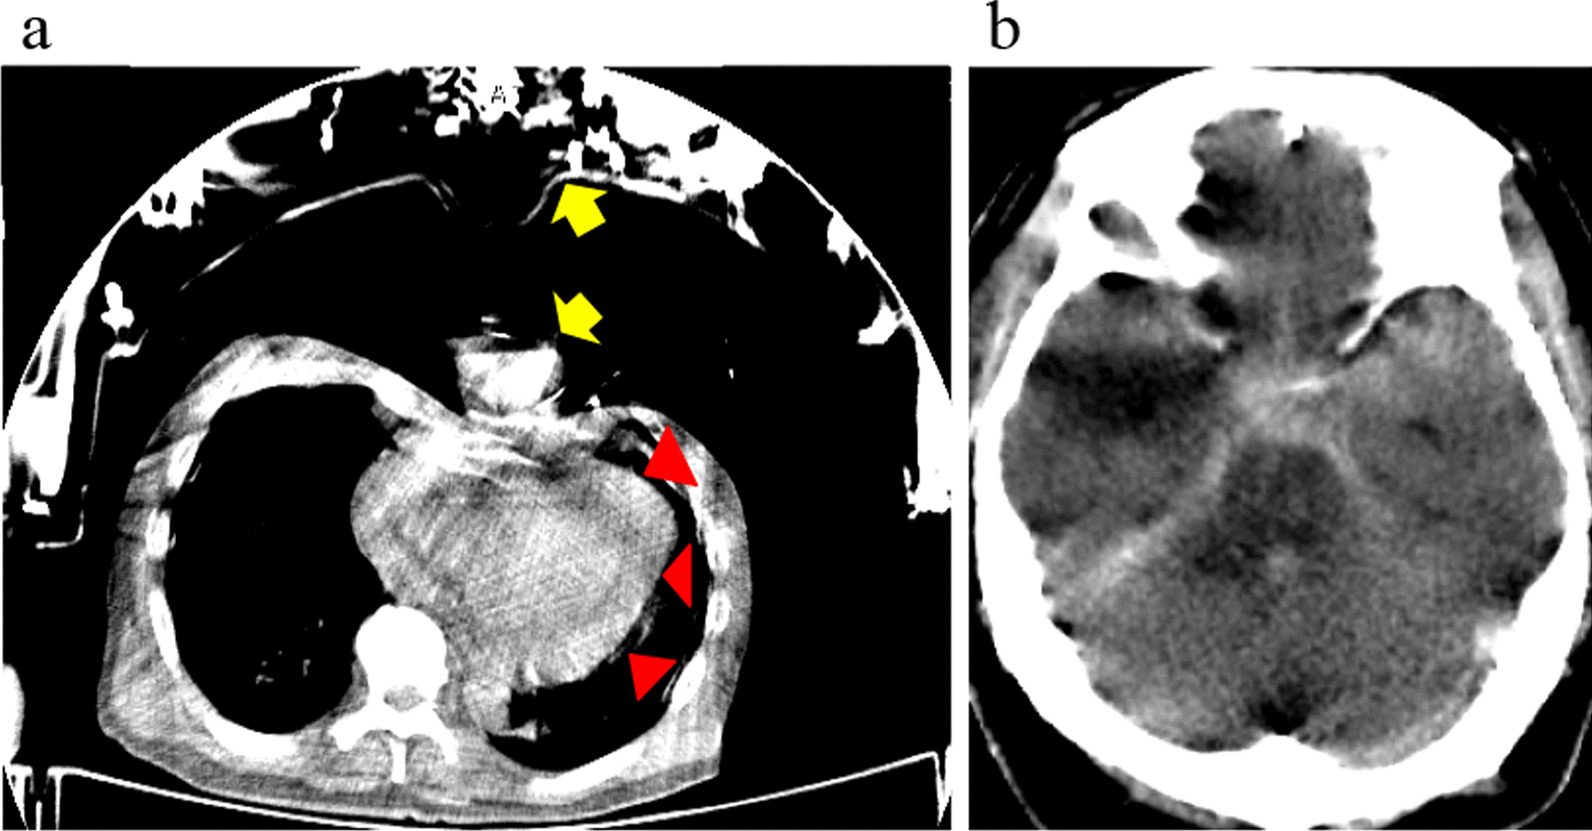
CT scan under mechanical CPR. a The CT scan shows pericardial effusion (triangle) and ACCD (arrows) (Case 5). b The CT scan shows subarachnoid haemorrhage (Case 7). *CT* Computed tomography, *CPR* Cardiopulmonary resuscitation, *ACCD* Automatic chest compression device
